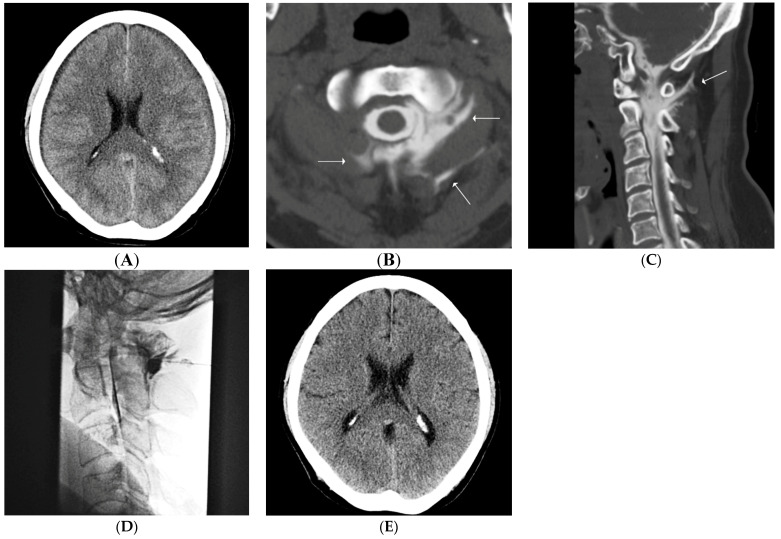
(A–E) Findings for a representative case involving a 53-year-old man with nontraumatic intracranial subdural hematoma (SDH). The patient presented with a chief complaint of headache not related to a specific posture. (A). Brain computed tomography (CT) image shows bilateral intracranial SDH. He was referred to us for CT myelography and epidural blood patch (EBP) the day after undergoing burr-hole trephination and hematoma removal. (B,C). Axial and sagittal CT myelography images show a large amount of cerebrospinal fluid leaks at the level of the C1/2 left extradural space (solid arrows). (D) EBP is performed at the C1/2 level. (E). Follow-up brain CT performed after 3 months shows no evidence of SDH.
